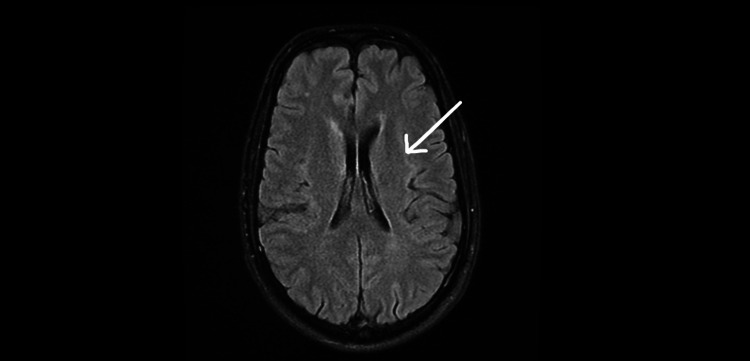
Axial MRI Brain showing a clear distinction between white and gray matter and no pathology MRI: magnetic resonance imaging
